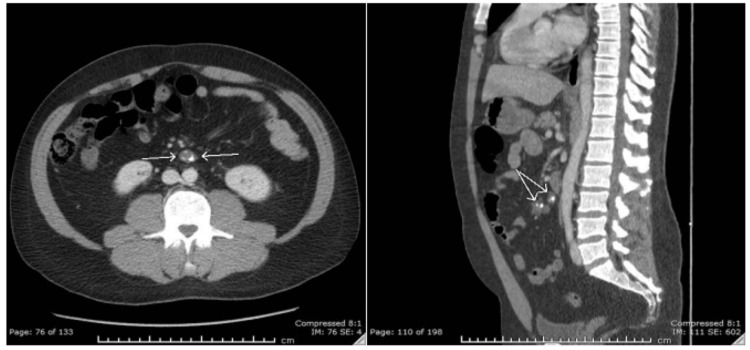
CT abdomen/pelvis Two mesenteric soft tissue foci with dystrophic calcifications (2.3 cm and 1.6 cm), possibly residual lymphoma or fat necrosis, are observed. White arrows on axial (left) and sagittal (right) views indicate calcified mesenteric foci. CT: computed tomography
